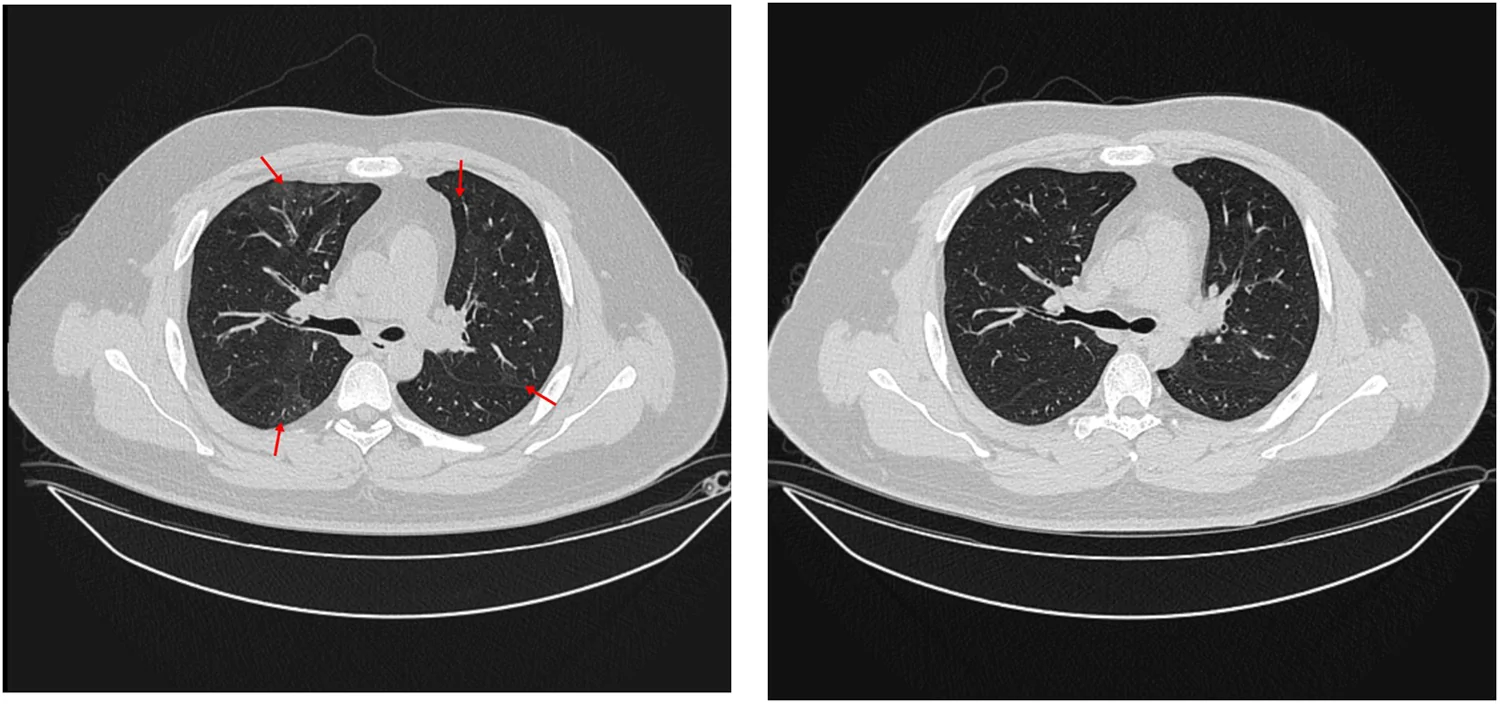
Chest computed tomography (CT) imaging. (Left) Initial CT on admission (Day 0) reveals bilateral multifocal patchy ground-glass and consolidative opacities (red arrows), accompanied by multiple small nodules in the upper lobes (identified on formal radiology department interpretation; below visual resolution on standard-view screenshots). (Right) Follow-up CT at 100 days demonstrates near-complete resolution of the bilateral patchy infiltrates, whereas the upper lobe nodules remain largely unchanged (per formal radiology department report).
